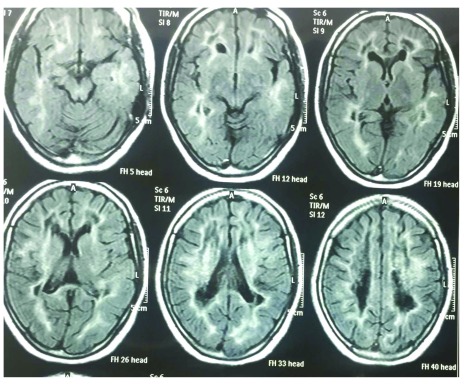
Cross-sectional view of T-2 weighted imaging with FLAIR demonstrating loss of cerebral white matter.
